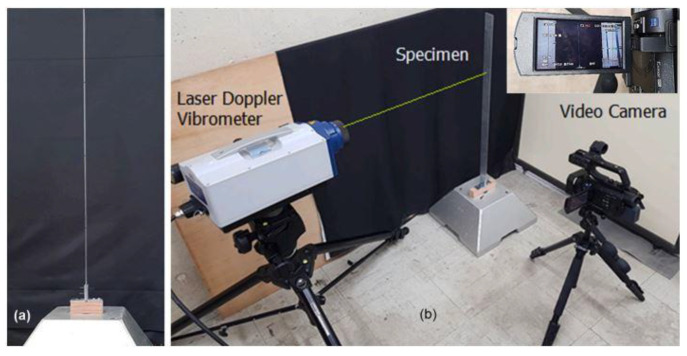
Cantilever beam: (a) initial configuration; (b) experimental setup featuring a video camera and a laser Doppler vibrometer (adapted from [66]).
